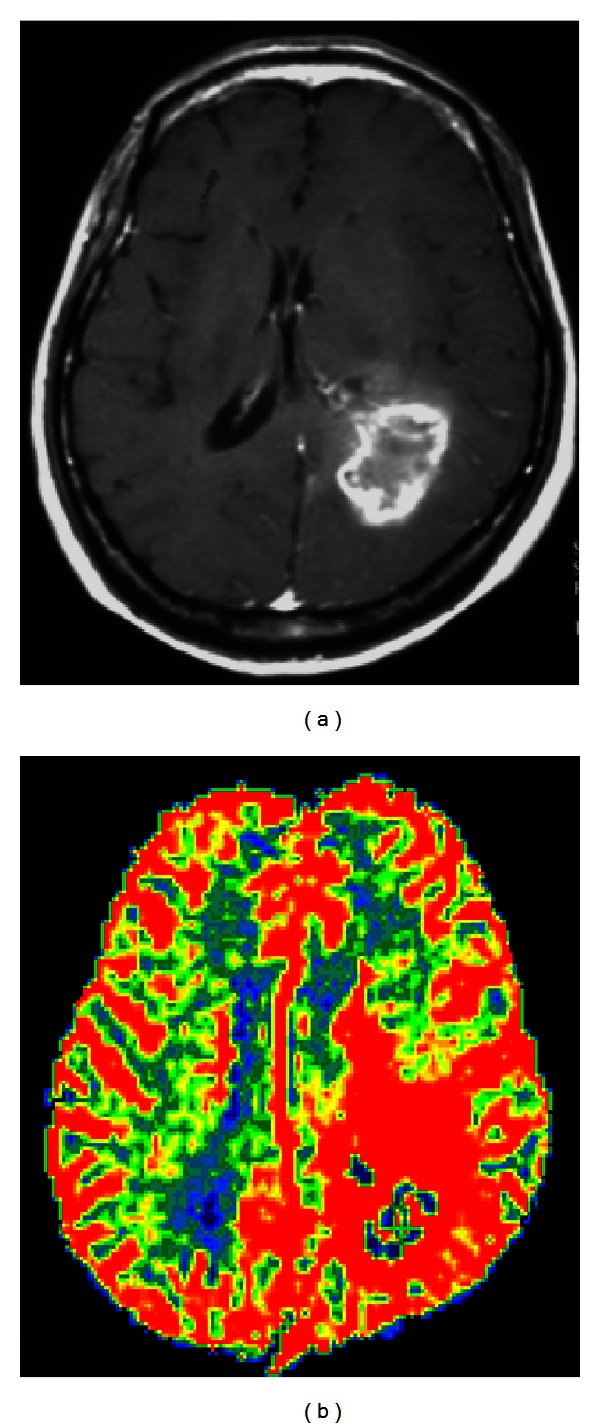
(a) Contrast-enhanced T1-weighted image demonstrates a ring-enhancing glioblastoma in the left parietal lobe with avid increase of rCBV (b) extending beyond the extent of the contrast enhancement.
